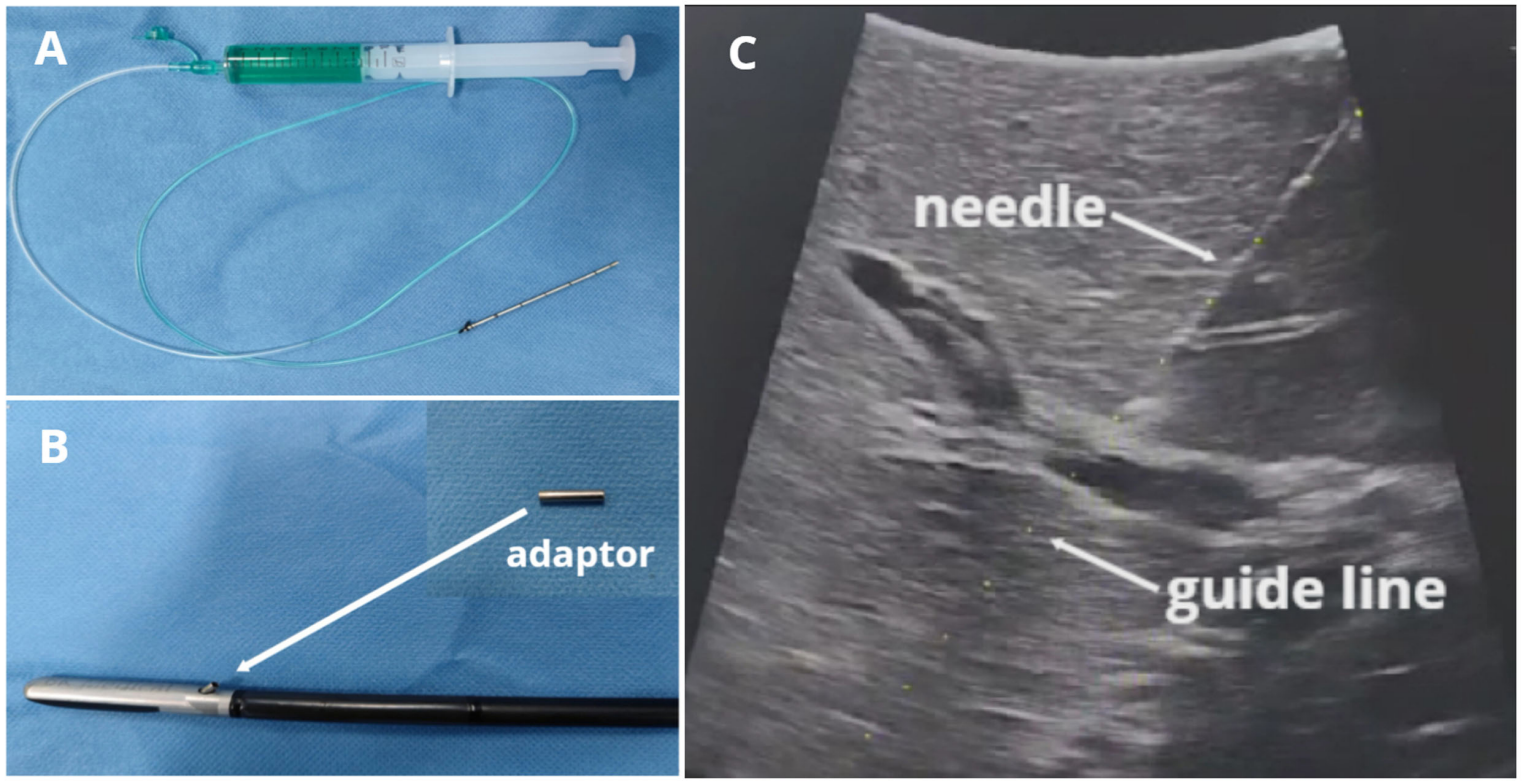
BK LUS-guided precise puncture with our customized needle and adaptor. (A) Puncture needle; (B) Laparoscopic ultrasound probe and adaptor; (C) Puncture needle and the guide line with the same path.
